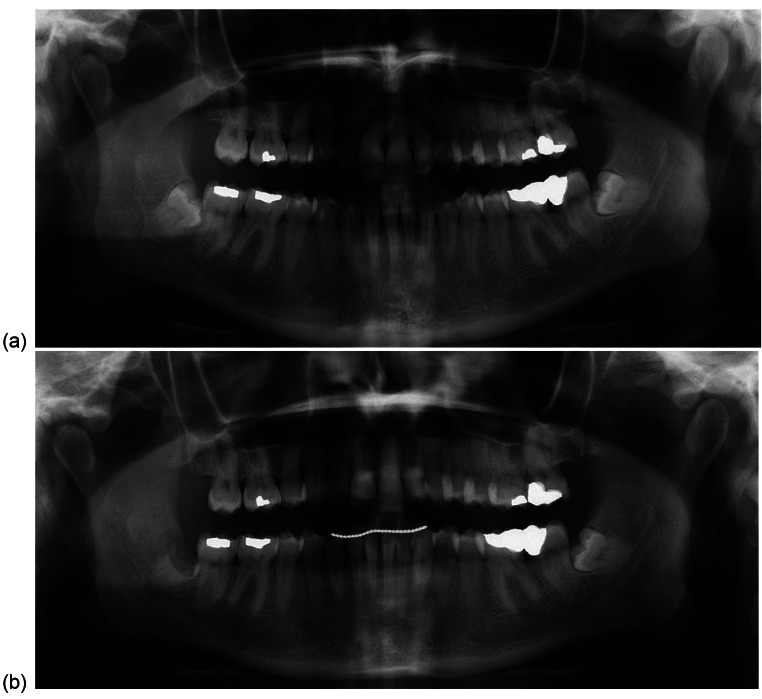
Pre-operative (a) and post-operative (b) orthopantomograms of Case 3
